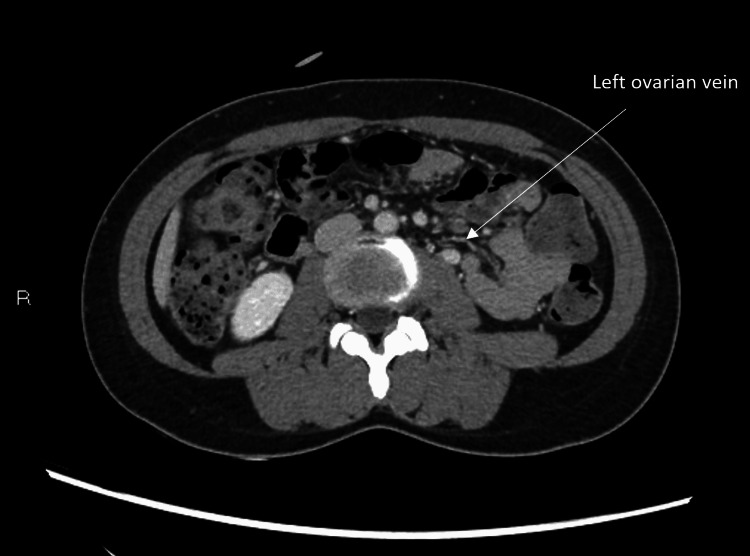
Axial CT images showing asymmetrically dilated left ovarian vein
